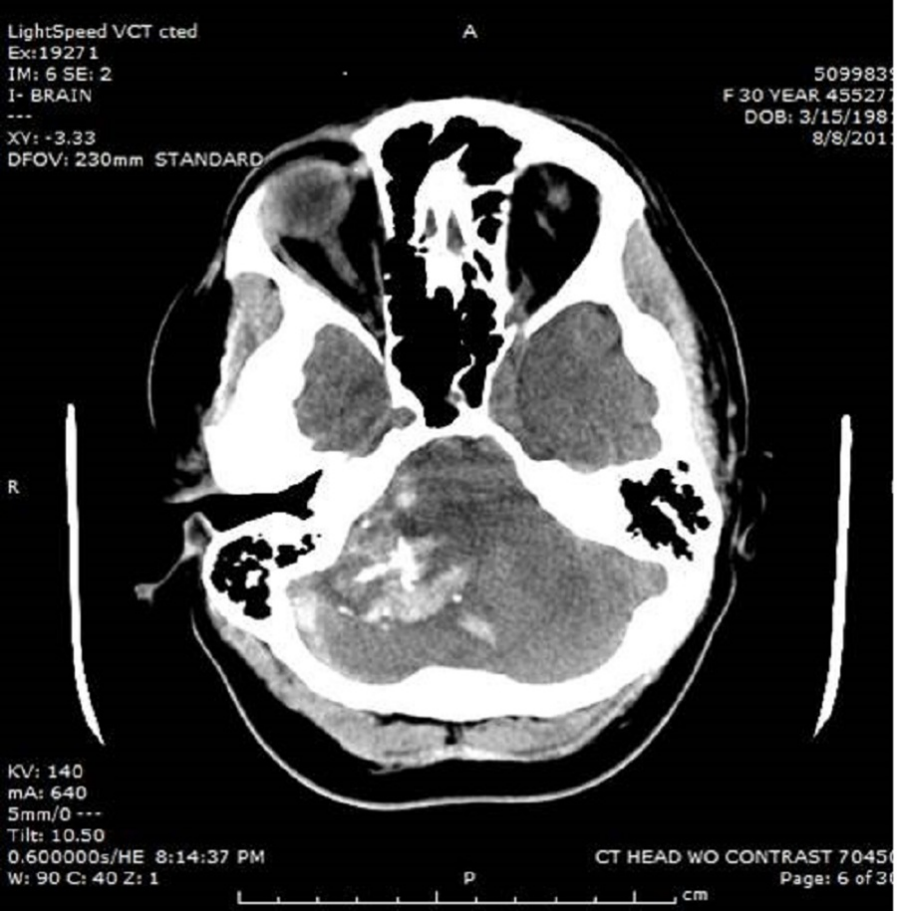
CT of the head revealing a right-sided cerebellar hemorrhagic calcified mass with effacement of the fourth ventricle.
